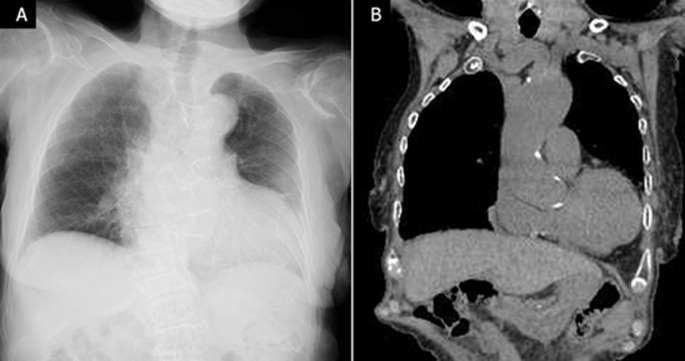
A) chest radiograph at 1-month follow-up examination (no recurrence is observed); B) computed tomography at 1-month follow-up examination (no recurrence is observed)
